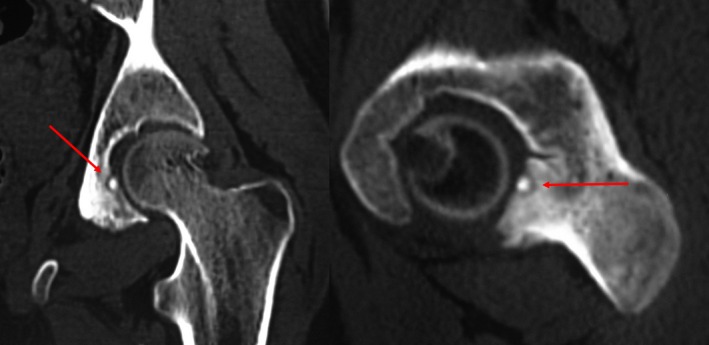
CT scan: bone sclerosis with a subchondral lytic lesion centered by the nidus
